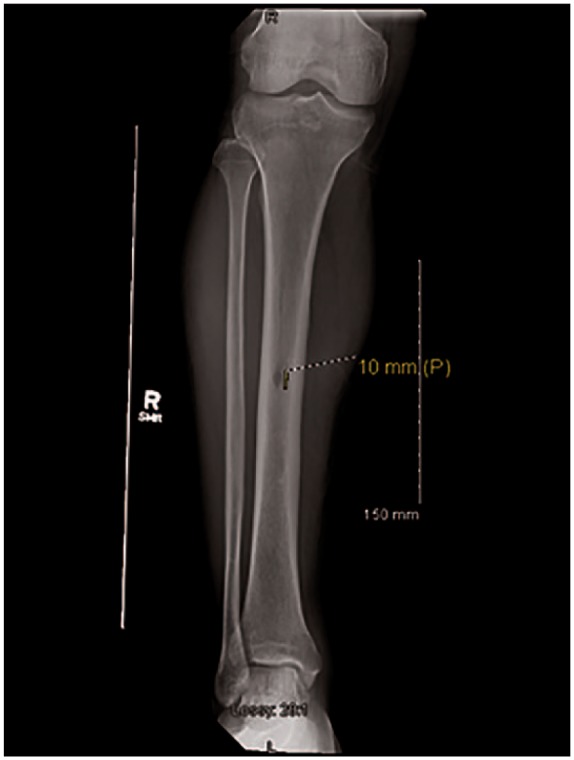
Tibial X-ray on admission for case 2.
